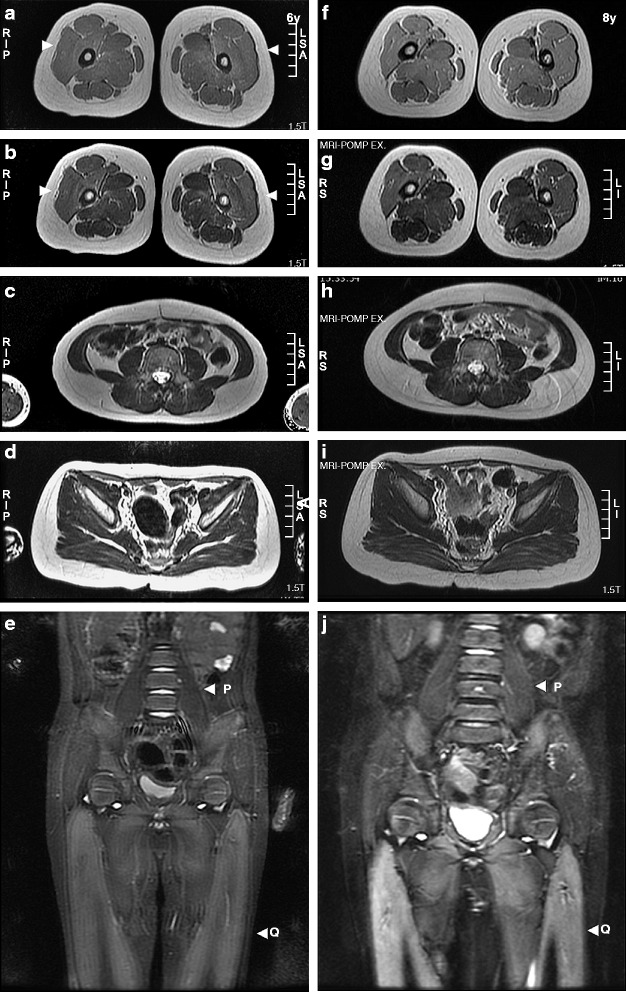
Sequential MRI studies in neonatally treated patients with IOPD. (a) Imaging of NBS4 was performed at 6 (left panel) and 8 years (right panel) of age. Changes were most severe in the thigh muscles (arrowheads), followed by the trunk and pelvic muscles. STIR revealed the rapid progression of hyperintensity over the quadriceps muscles. (*a*, *f*) Thigh T1WIs, (*b*, *g*) thigh T2WIs, (*c*, *h*) trunk T2WIs, (*d*, *i*) pelvic T2WIs, and (*e*, *j*) coronal STIR images. Q: quadriceps muscles; P: psoas muscles. (b) Similar thigh T2WI changes in patients examined at 5 (*a*–*c*) and 8 years (*d*–*f*) of age. T2WI signal changes and muscle atrophy were prominent, particularly over the quadriceps muscles in the IOPD patients but not in the LOPD patients who were administered rhGAA therapy: (*a*, *d*) NBS2, (*b*, *e*) NBS3, and (*c*, *f*) NBSL2. (c) Variable degrees of thigh T2WI changes in patients examined at 3 (*a*–*c*) and 5 years (*d*–*f*) of age. T2 signal changes over the quadriceps muscles and increased intramuscular fat were prominent the IOPD patients but not in the LOPD patients who were administered rhGAA therapy: (*a*, *d*) NBS5, (*b*, *e*) NBS6, and (*c*, *f*) NBSL6
